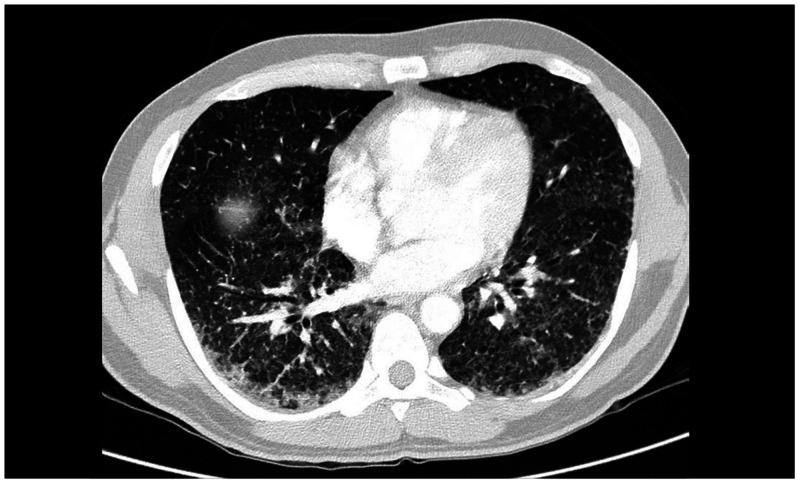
Axial view. Contrasted chest computed tomography scan showing multiple centrilobular micronodules, with a budding tree pattern and ground glass.
